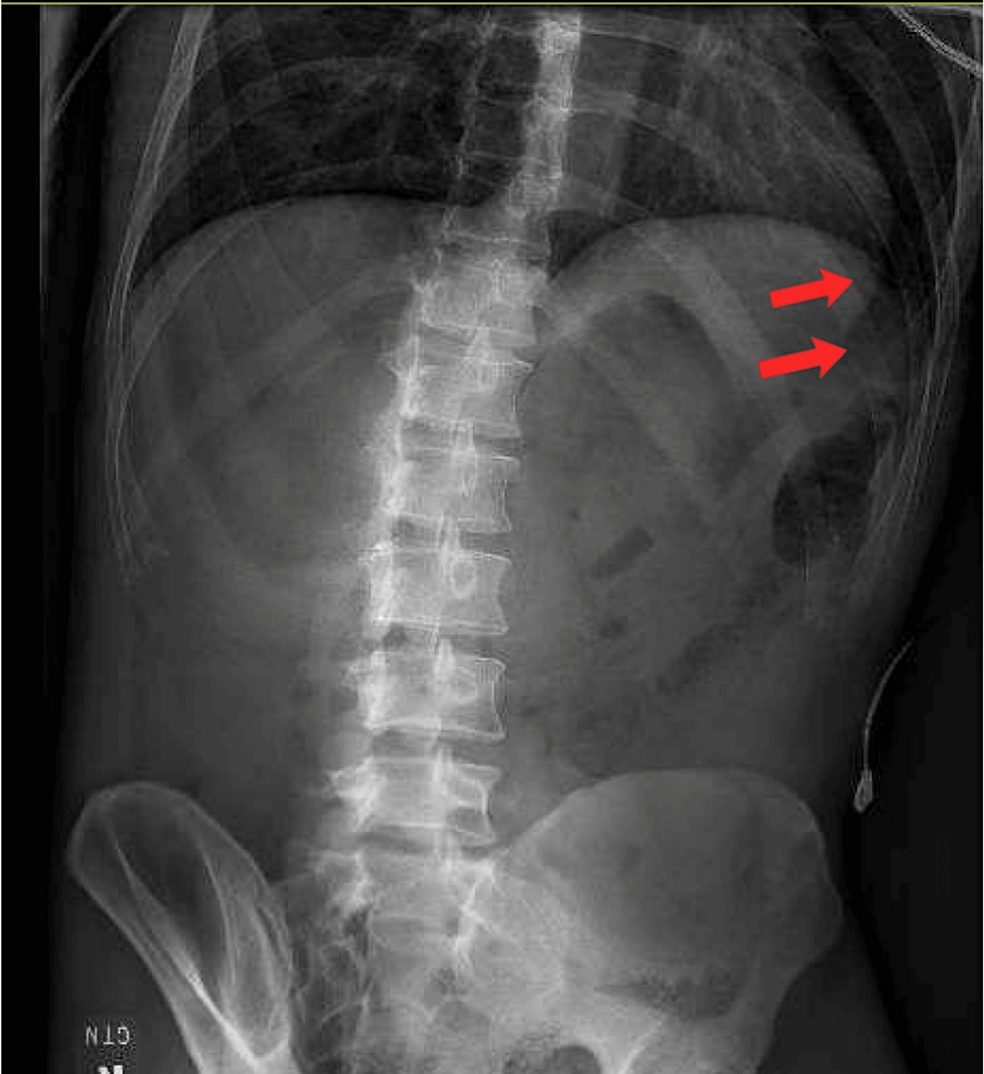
Abdominal X-ray indicating potential extraluminal gas The red arrows indicate an area of lucency in the upper abdomen, raising suspicion for extraluminal gas.
